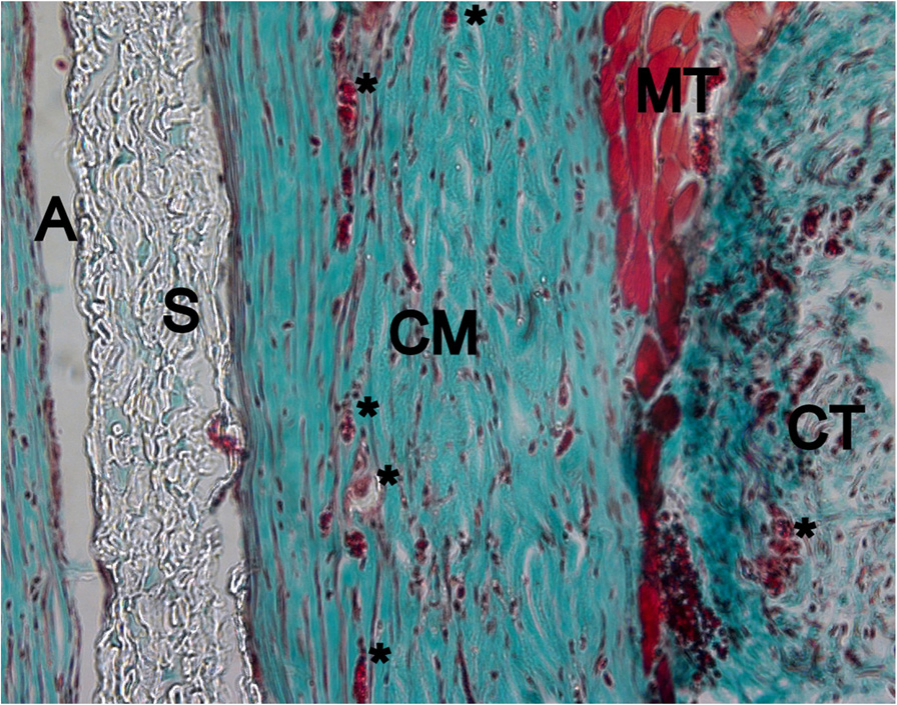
Complete biodegradation of ND after 12 weeks healing period (Masson Goldner, Original magn. 200×). CM: collagen matrix, MT: muscle tissue, *: blood vessel, CT: connective tissue, S: spacer, A: artefact.
